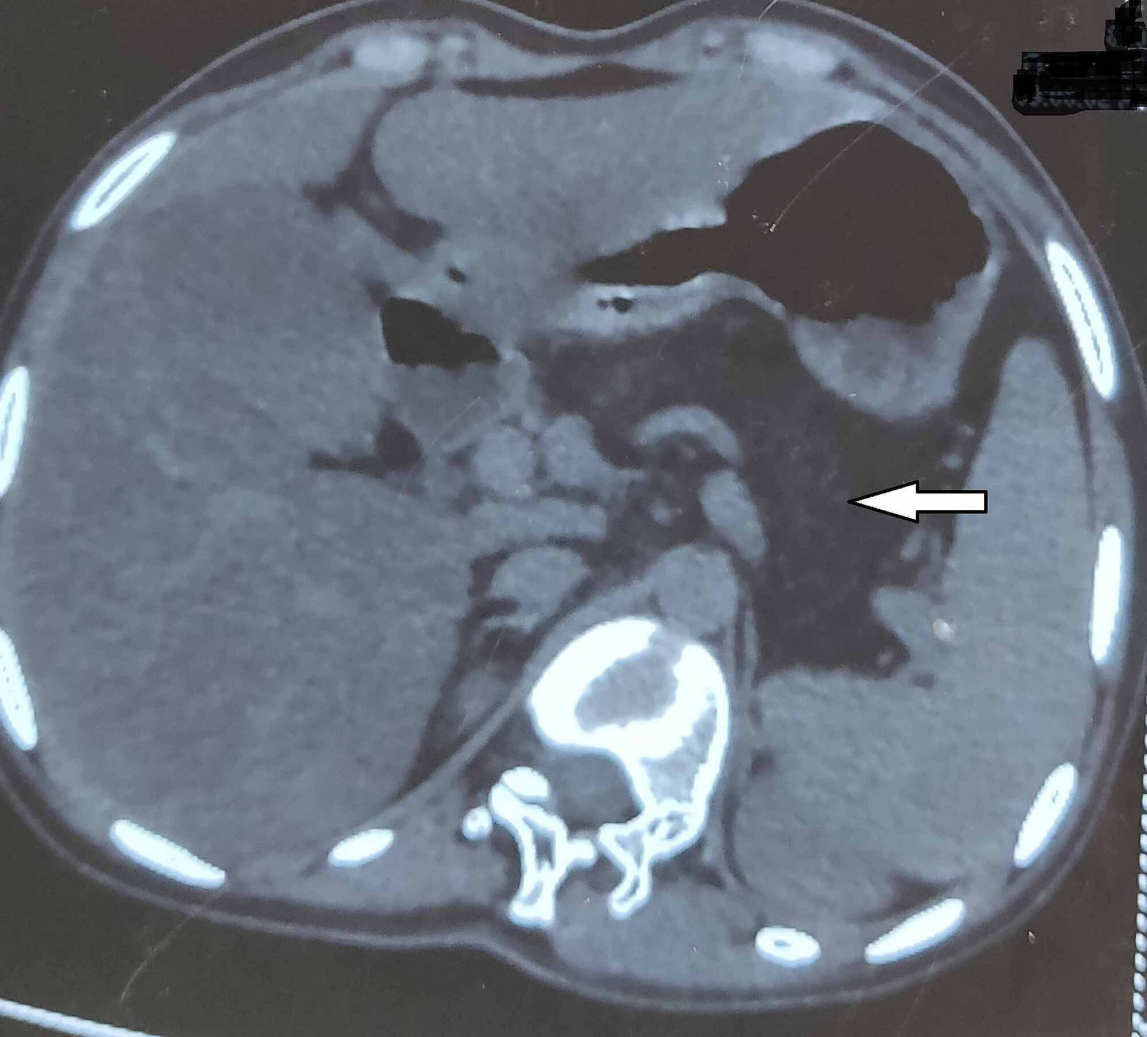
CT of the abdomen showing total pancreatic lipomatosis CT: computed tomography
